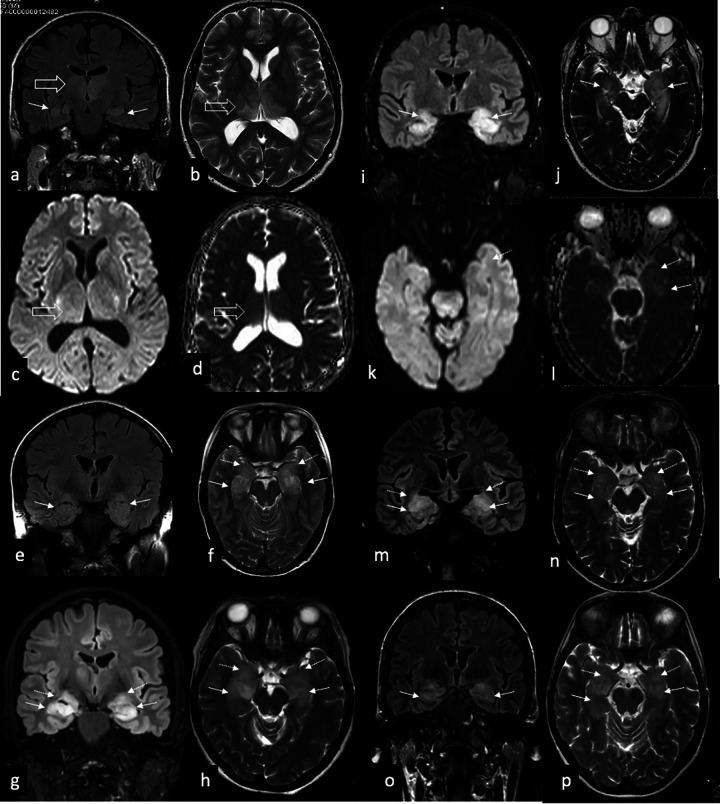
Similar MRI imaging findings in different patients:. a-d) index patient IV-1 with hyperintensity of the hippocampi on coronal FLAIR (arrows, a) and thalami on axial T2w [open arrow, (b) without restricted diffusion on DWI (c) and corresponding ADC (d) in the thalami e-f] coronal FLAIR (e) and axial T2w (f) of patient V-2 with bilateral hyperintensity and swelling of hippocampi (arrow) and amygdala (dotted arrow) g-h) coronal FLAIR (g) and axial T2w (h) of patient IV-7 with bilateral hyperintensity and swelling of hippocampi (arrow) and amygdala (dotted arrow) i-l) coronal FLAIR (i) and axial T2w (j) of patient IV-21 with hyperintensity and swelling of hippocampi (arrow) and amygdala (dotted arrow), left more than right without restricted diffusion in the mesial temporal lobe on DWI (k) and corresponding ADC (l) m-n) coronal FLAIR (m) and axial T2w (n) of patient IV-20 with bilateral hyperintensity and swelling of hippocampi (arrow) and amygdala (dotted arrow) o-p) coronal FLAIR (o) and axial T2w (p) of patient V-23 with bilateral hyperintensity and swelling of hippocampi (arrow) and amygdala (dotted arrow).
